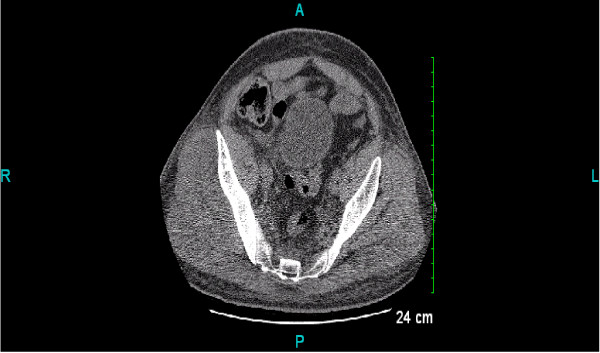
CT scan of the pelvis - Fullness of the right gluteal maximus and medius muscles, possibly secondary to edema or inflammation or compartment syndrome.
